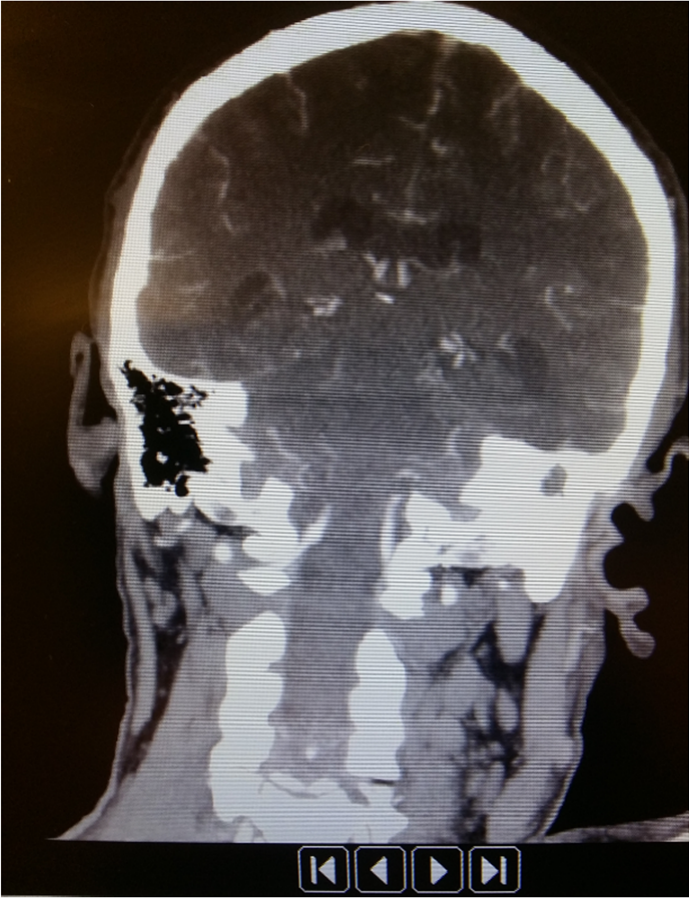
Skull CT: coronal slice of brain with left side mastoiditis
